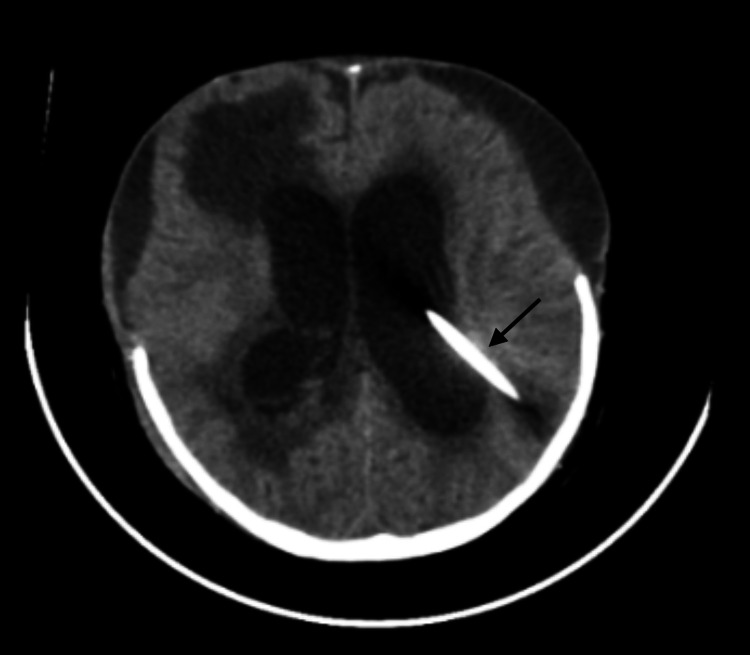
MRI showing VP shunting was done following which she developed CSF otorrhea in the postoperative period.
